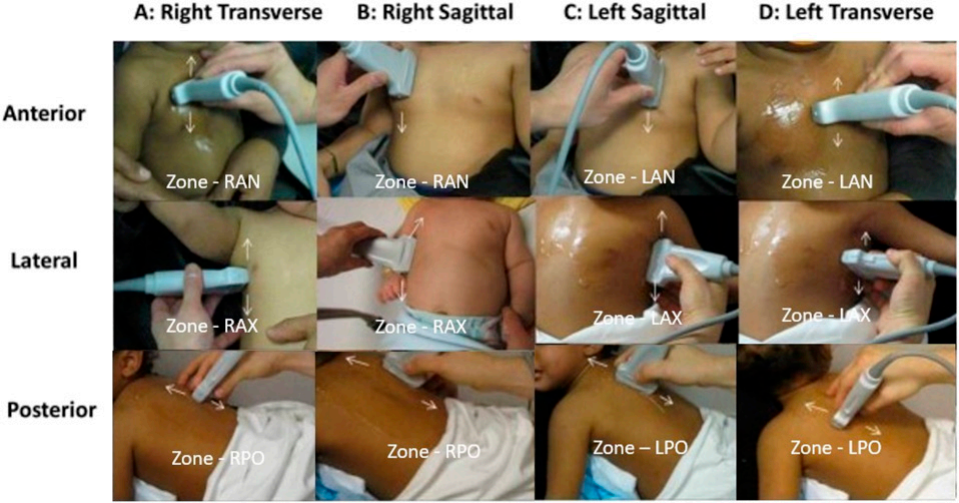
Six zone, 12 view lung ultrasound scan protocol.^14^
